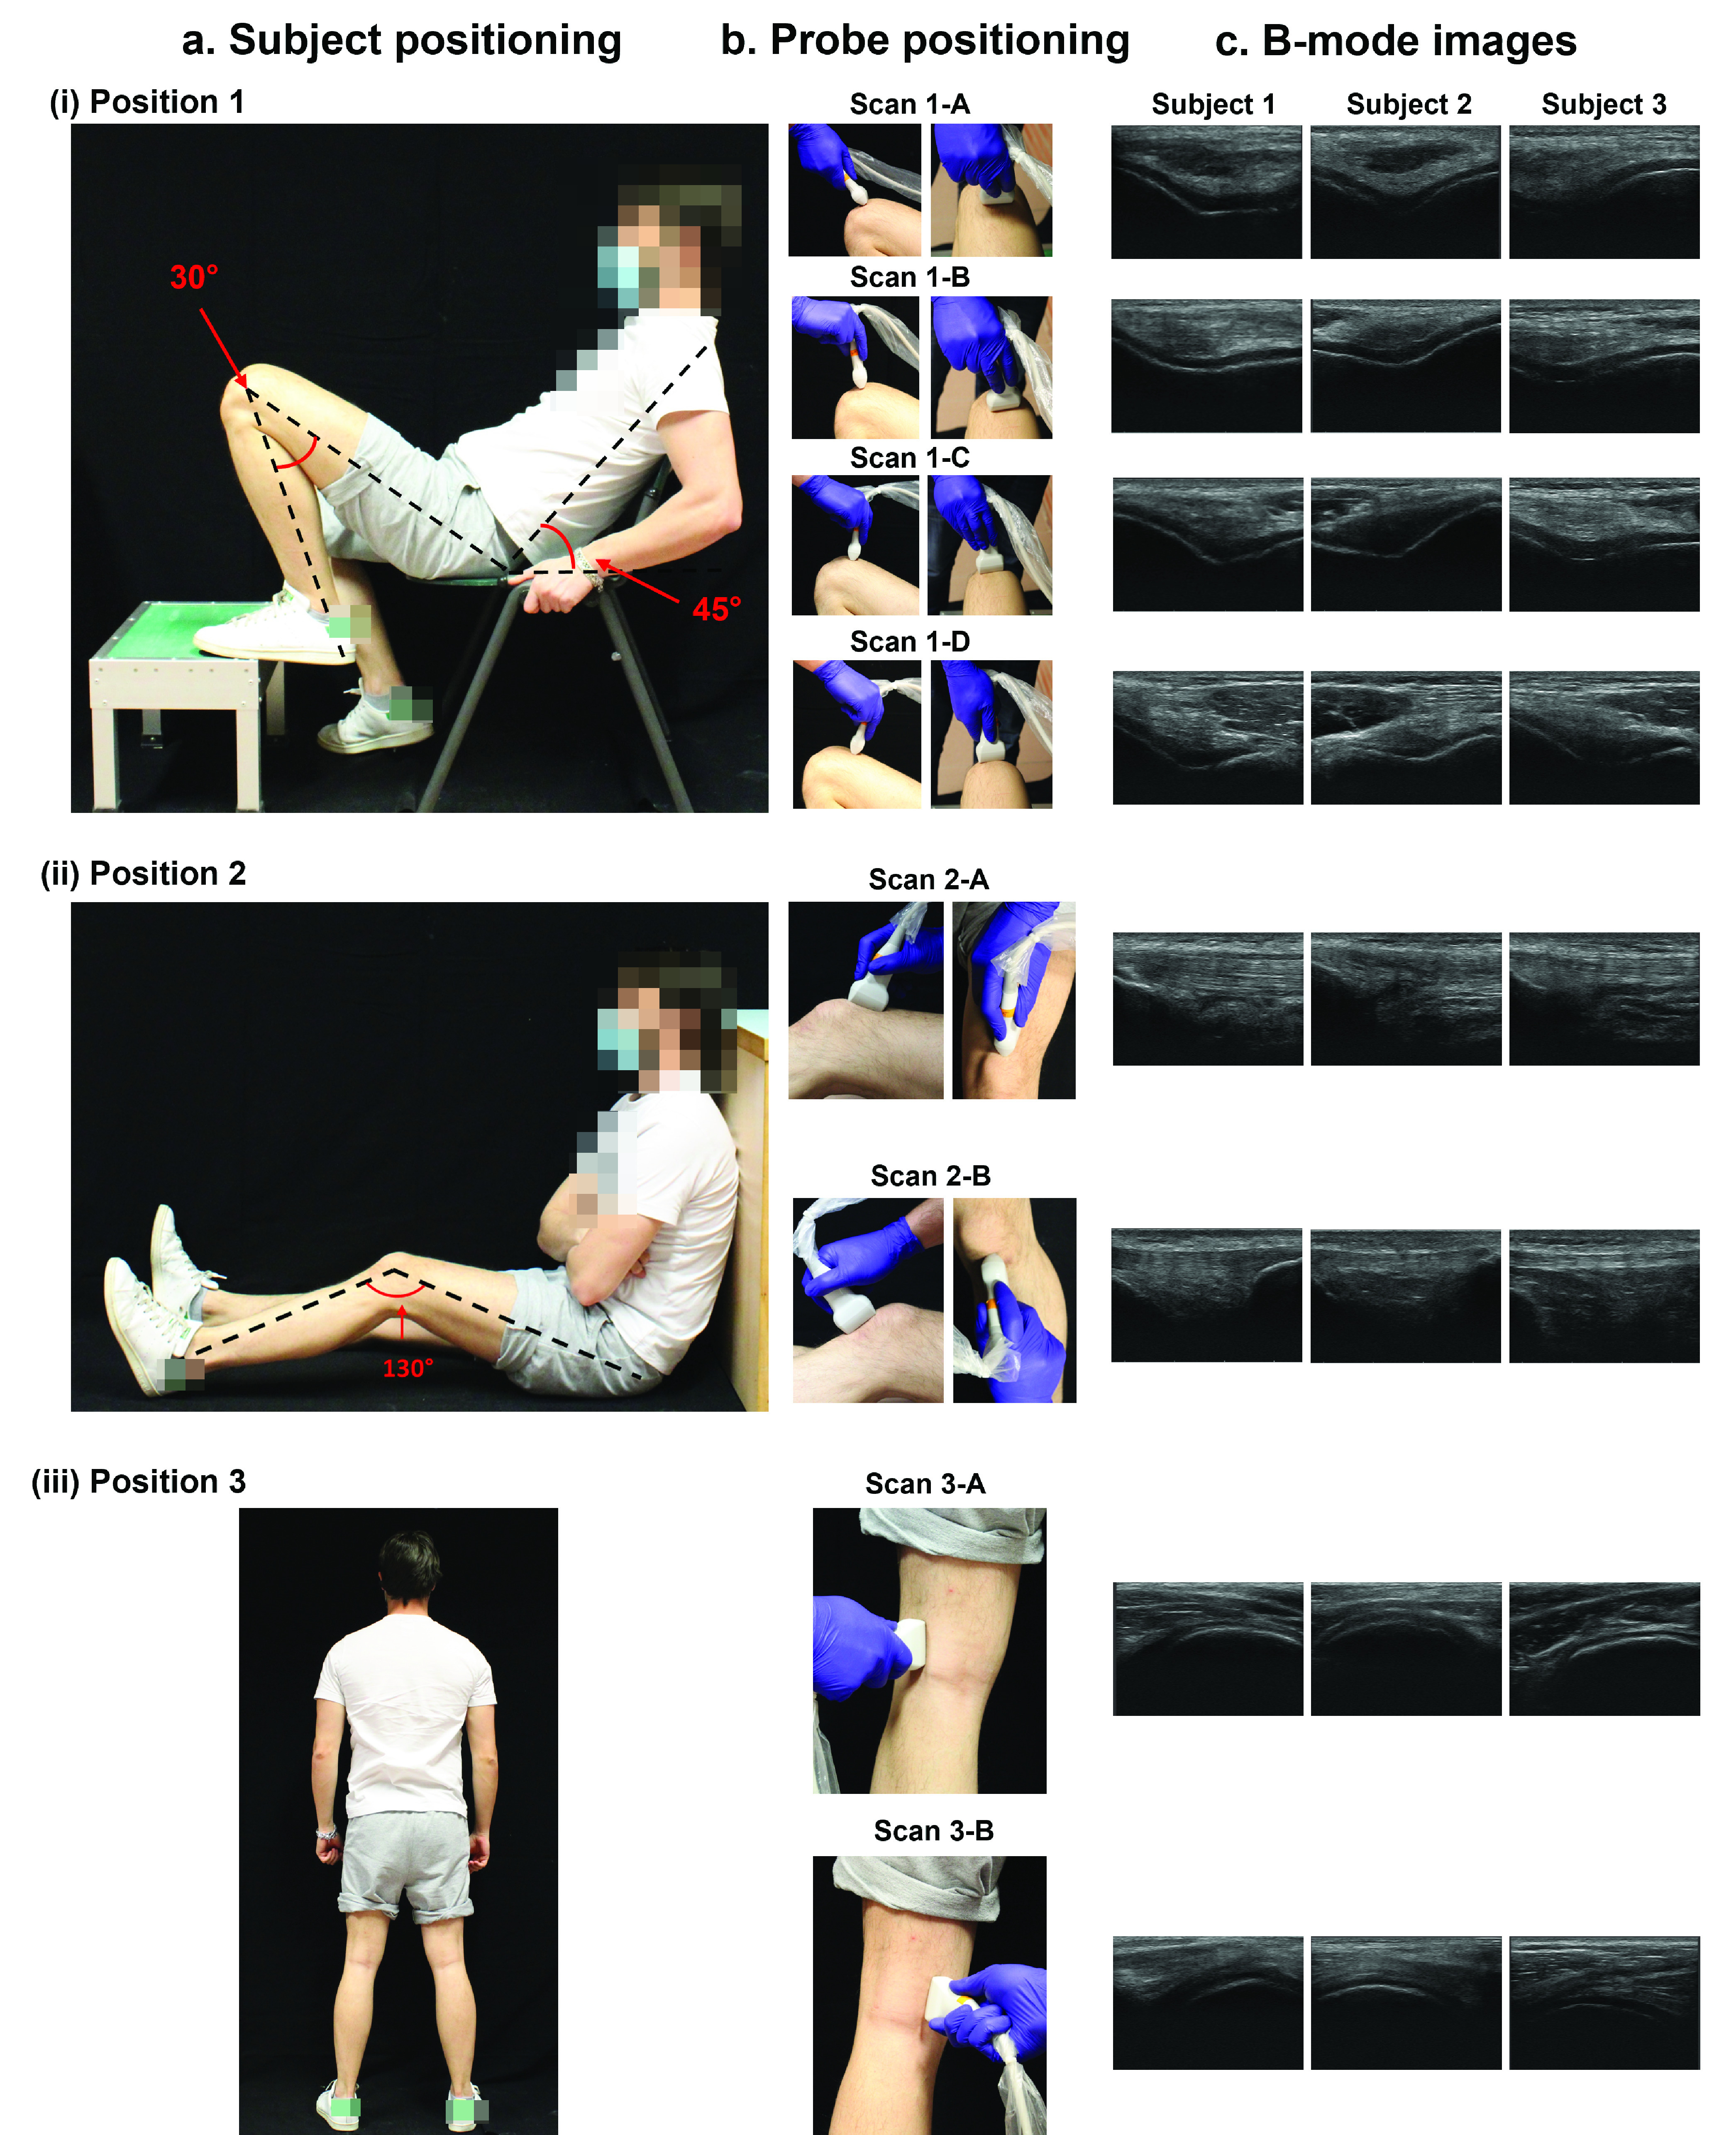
Clinicians’ indications on patient and ultrasound probe positioning for US imaging of the cartilage. In panel a-(i) the subject is seated on a chair in Position 1 and different scanning planes are analyzed: Scan 1-A, Scan 1-B, Scan 1-C and Scan 1-D (b-(i)), to assess the anterior cartilage (c-(i)). In panel a-(ii), the subject is seated on the floor in Position 2 and two scanning planes are analyzed: Scan 2-A and Scan 2-B (b-(ii)), to visualize the synovium and the Hoffa’s adipose body (c-(ii)). In panel a-(iii) the subject is standing in Position 3 and two scanning regions are analyzed: Scan 3-A and Scan 3-B (b-(iii)), to assess the posterior cartilage (c-(iii)).
